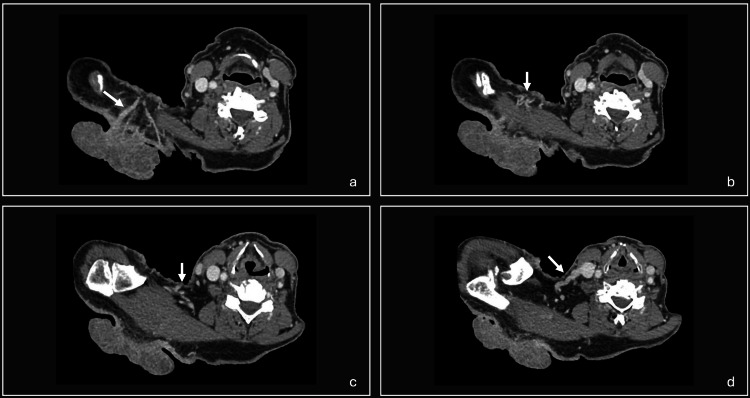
CT of the neck with IV contrast. (a) A robust vascularization with large draining veins extending from the lesion. (b and c) Draining veins from the lesion pooling in a central venous confluence. (d) Draining veins from the lesion extending to the right internal jugular vein.
